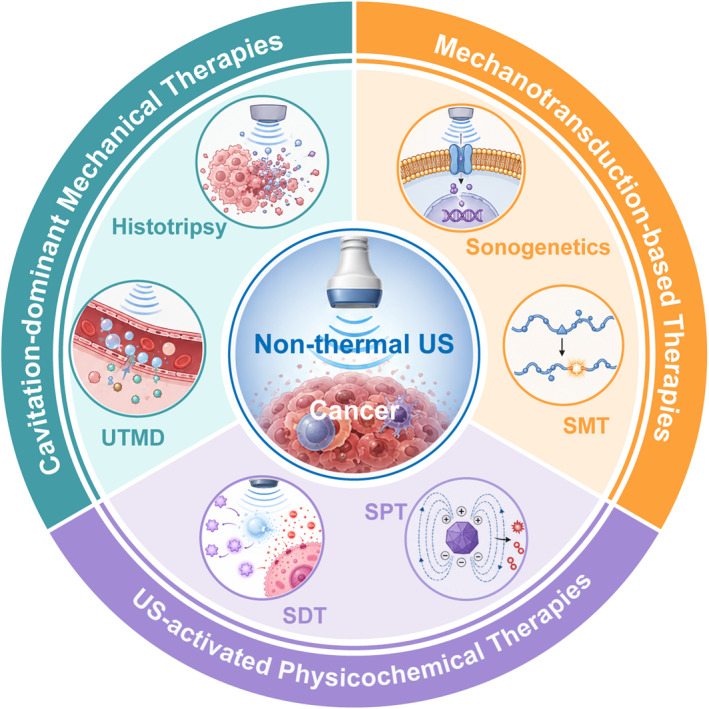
Mechanism‐guided classification of non‐thermal ultrasound‐based cancer therapies according to parameter‐dependent bioeffects.
